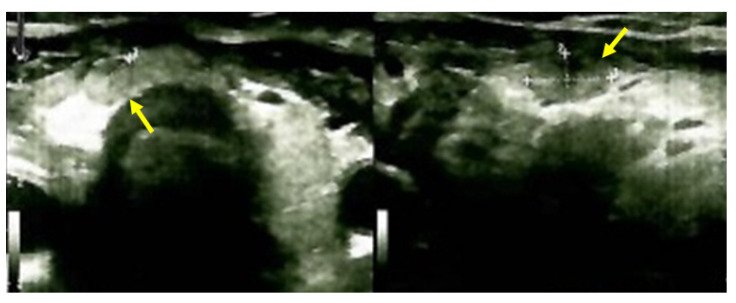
Neck ultrasound after right subtotal thyroidectomy and right inferior parathyroidectomy: an isoechoic and relatively homogeneous thyroid pattern with an intensely vascularized, inhomogeneous nodule of 0.91 by 0.54 by 0.78 cm (yellow arrow) at the right lobe-isthmus junction, which required follow-up; the images did not suggest another parathyroid tumor was present.
